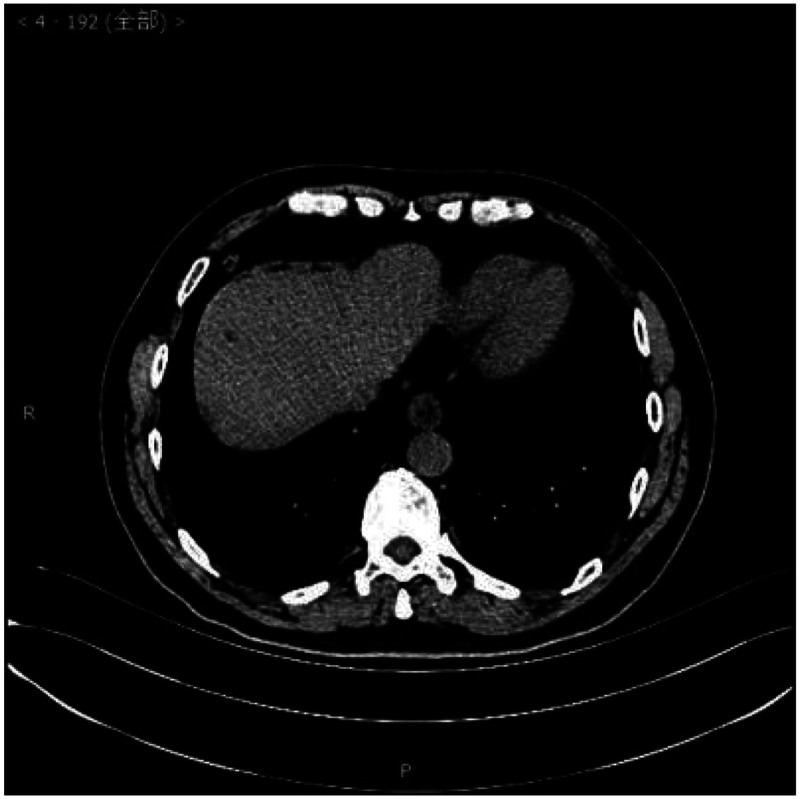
Chest computed tomography scan.
